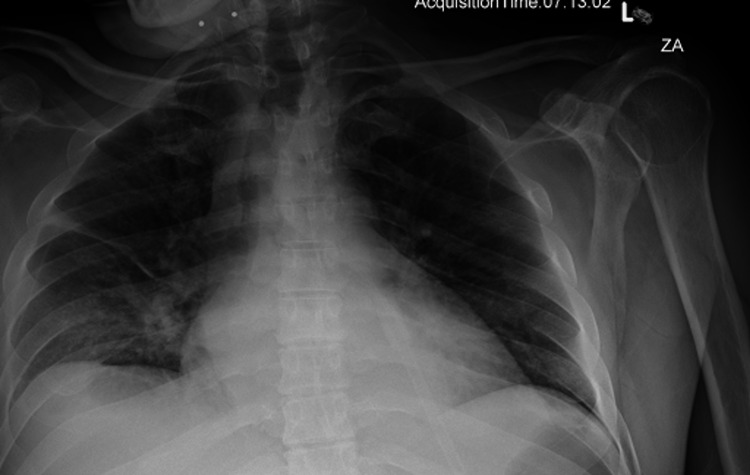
Chest X-ray Chest X-ray showing a right mid-lower lung zone opacities could represent infection and/or aspiration.
